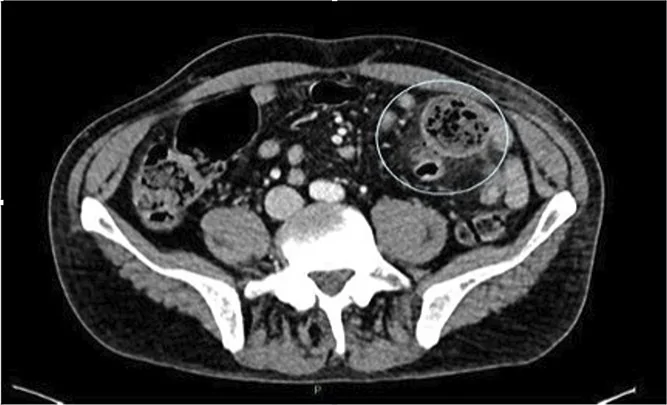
Contrast-enhanced CT abdomen demonstrating distal ileal mural thickening with localized extraluminal gas, consistent with contained perforation.
